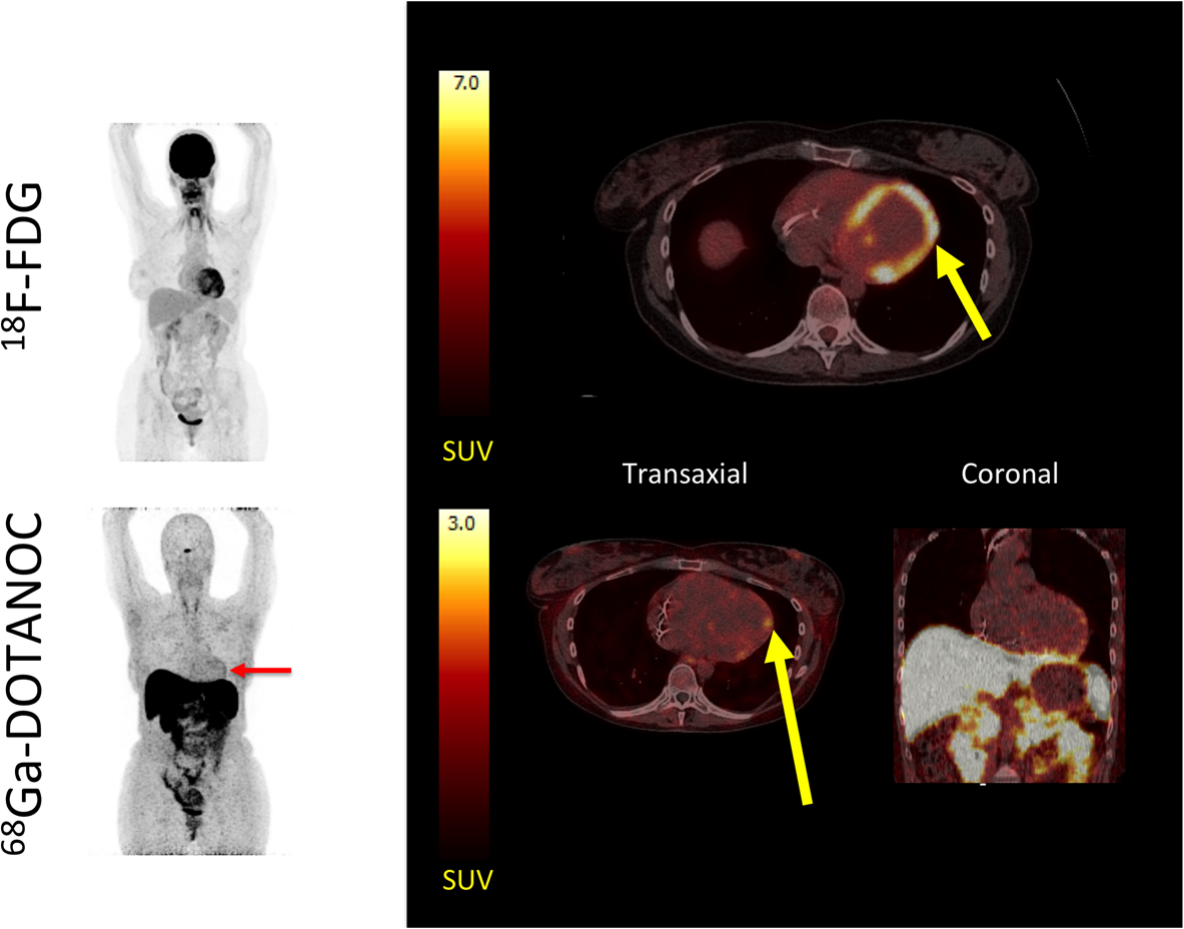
Patient with CS. ^18^F-FDG PET/CT images were rated as inconclusive due to insufficiently suppressed physiological ^18^F-FDG uptake. *Left panel*: MIPs showing ^18^F-FDG PET/CT (*upper*) and ^68^Ga-DOTANOC PET/CT (*lower*) of patient no. 3. ^68^Ga-DOTANOC accumulation can be seen in the anterior and lateral wall (*red arrow*). *Right upper panel*: transaxial slices of ^18^F-FDG showing focal on diffuse ^18^F-FDG uptake considered inconclusive by a majority of readers. *Right lower panel*: ^68^Ga-DOTANOC accumulation (*yellow arrow*) is seen in the anterolateral and lateral wall (SUVmax 2.35, target-to-background 1.56). In the coronal view, it is evident that spill-in activity from the liver may obscure inferior lesions
